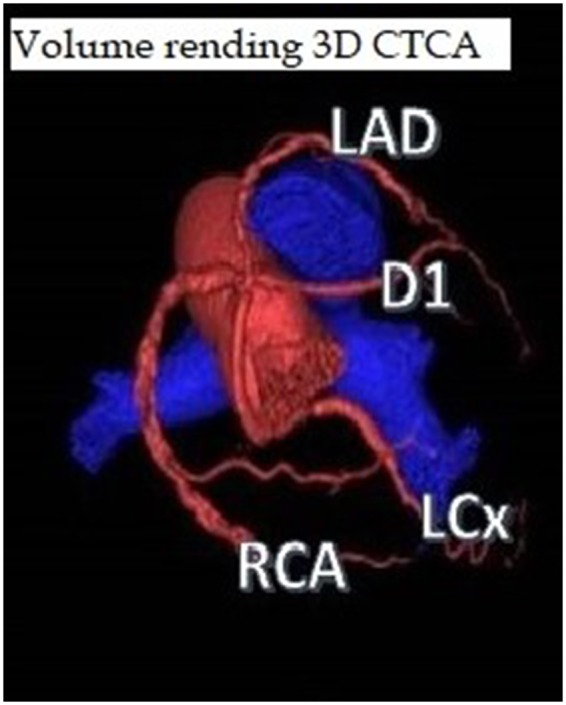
Volume rending three-dimensional computed tomography coronary angiogram shows single coronary ostium arising from the right with three abnormal courses of coronary arteries and only right coronary artery was found to have a normal course. Left circumflex coronary artery was found to have retro-aortic course, left anterior descending artery had pre-pulmonic course, and first diagonal had a sub-pulmonic (septal) course.
